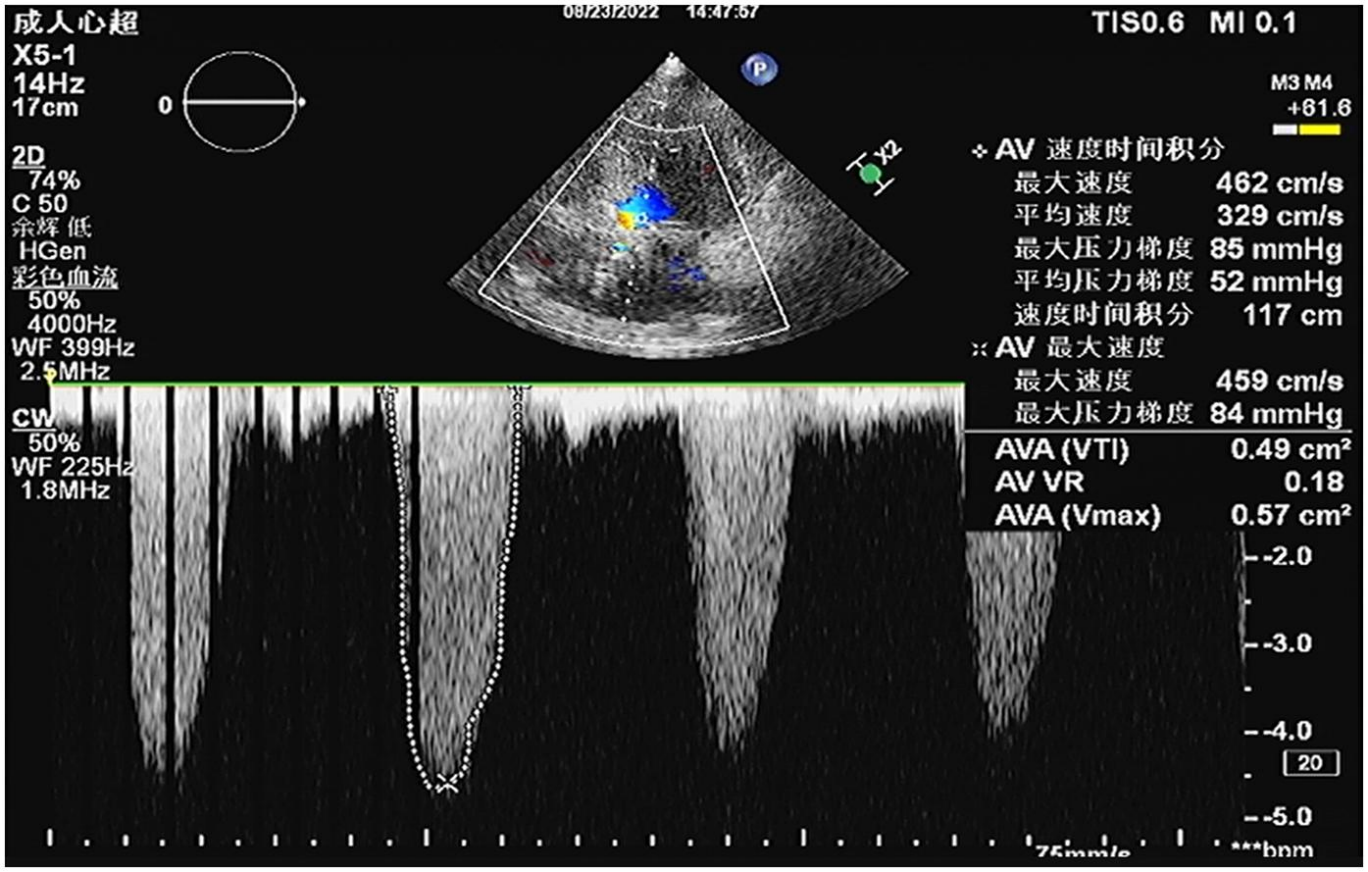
Preoperative transthoracic echocardiography (TTE) revealed severe aortic valve stenosis with a peak transvalvular velocity of 4.6 m/s, peak pressure gradient (PG) of 85 mmHg, mean pressure gradient (mPG) of 52 mmHg, aortic valve area (AVA) by velocity-time integral (VTI) of 0.49 cm^2^, and aortic regurgitant area of 4.6 cm^2^.
